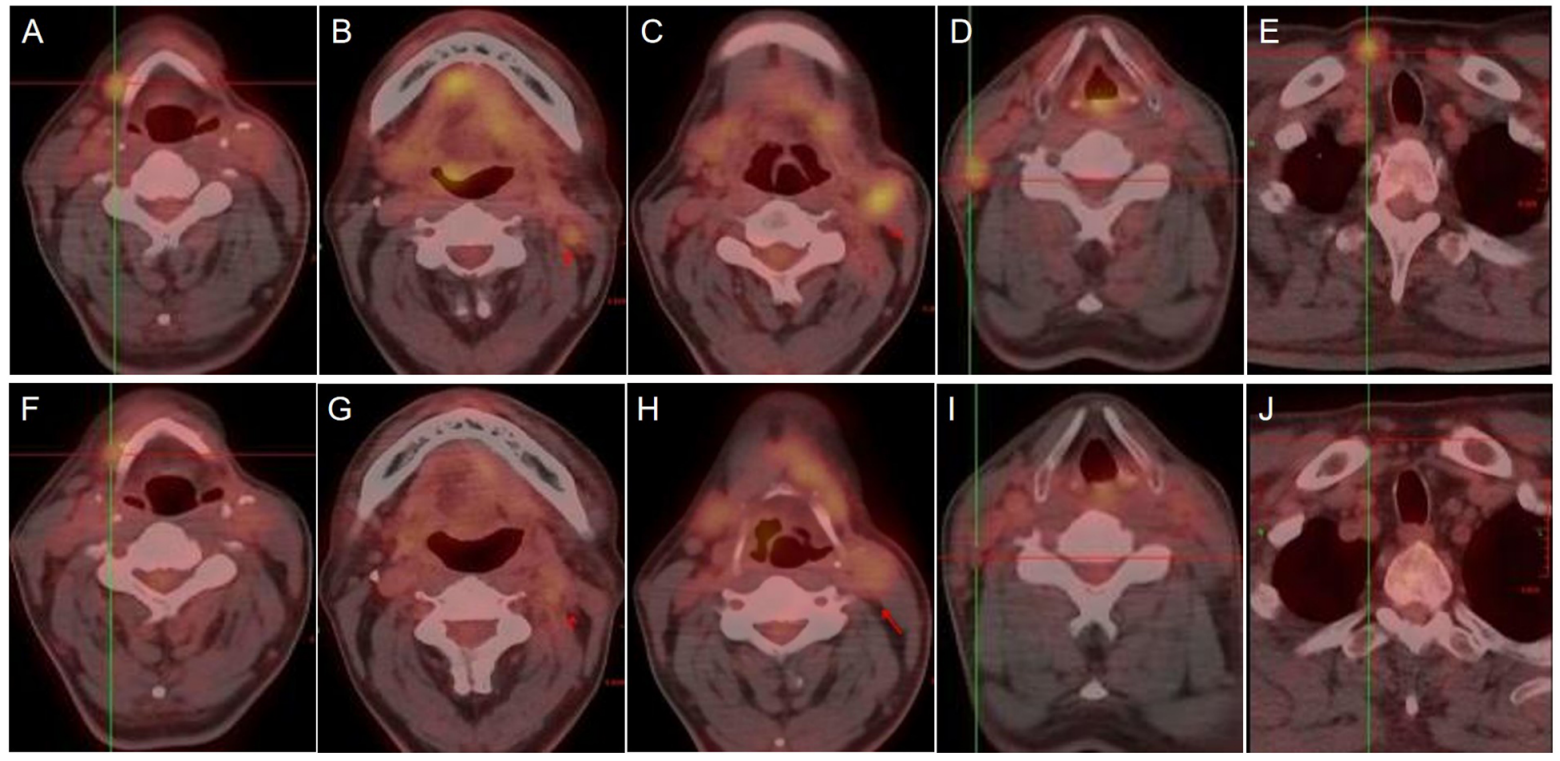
PET/CT images showing cervical lymph nodes before (A-E) and after (F-J) treatment with proton therapy.
